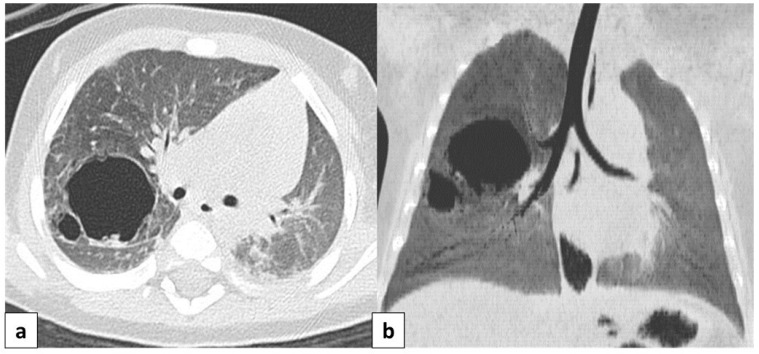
Axial CT scan performed on a 4 month old female patient, depicting a type 1 CPAM. In image (a), it is possible to appreciate a large (>2 cm) air-filled cyst, surrounded by other multiple smaller cysts. Additionally, in image (b), a coronal CT MinIP reconstruction of the same patient is shown, which excludes communication with the tracheo-bronchial tree.
